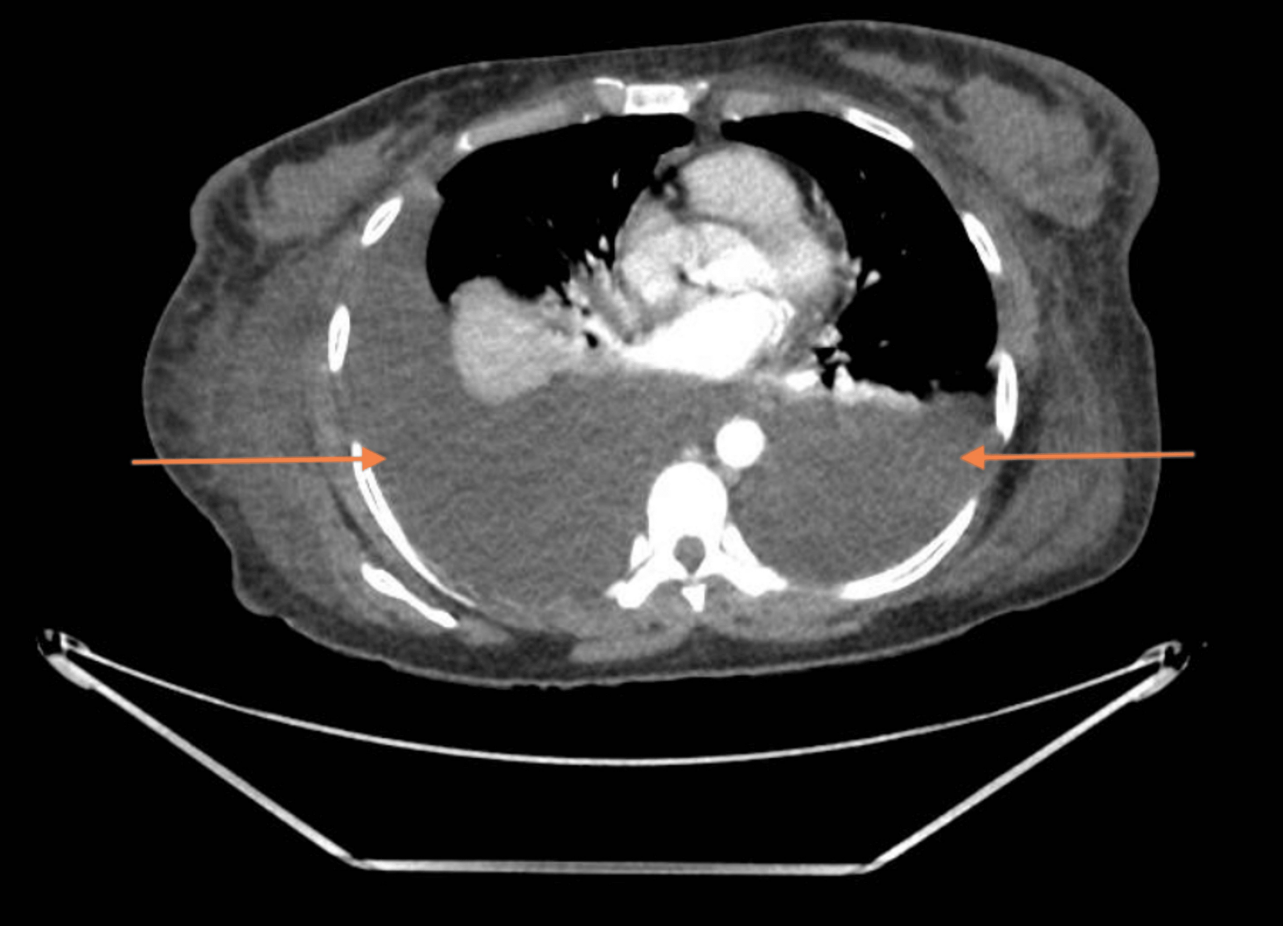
CT chest cross slice from CT chest abdomen and pelvis. Significant bilateral pleural effusions delineated by arrows.
